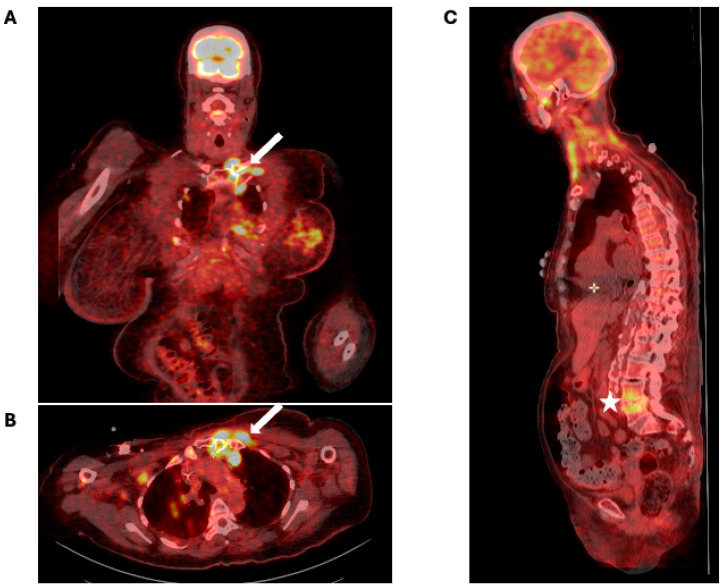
Sternoclavicular and spine OASGs detected by FDG-PET/CT. (A,B): Arthritis of the left sternoclavicular joint (white arrow; (A): coronal section, (B): axial section); (C): spondylodiscitis at the L4–L5 level (white star).
